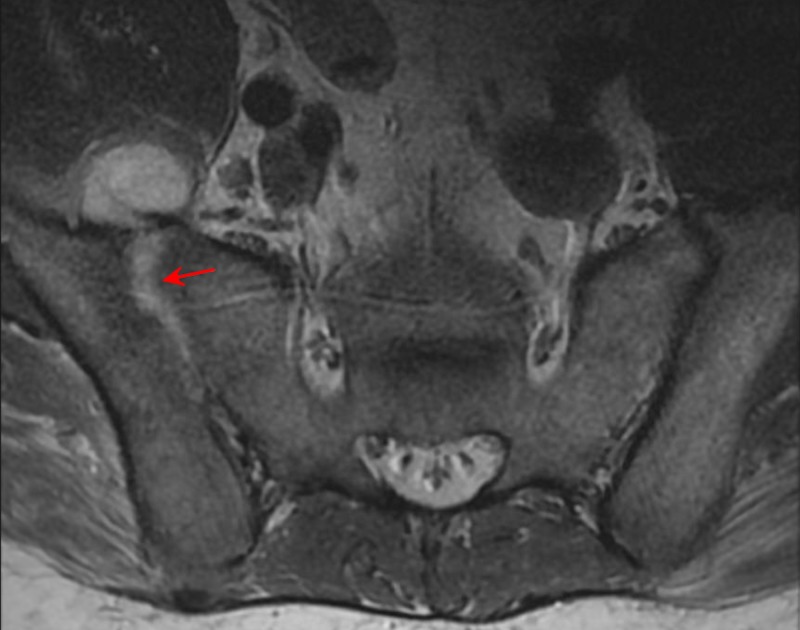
Preoperative magnetic resonance imaging (MRI) displaying right sacroiliac joint effusion with edema
